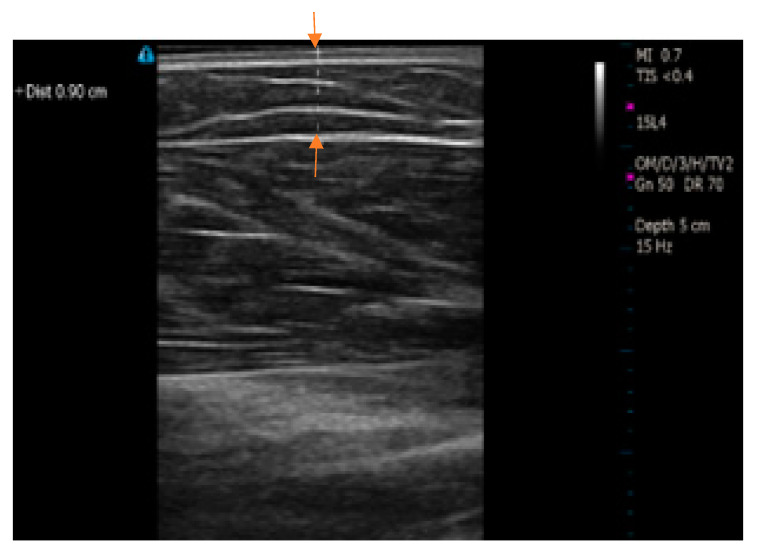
Ultrasound imaging for the measurement of subcutaneous fat thickness. Arrows show the measured skin-fat thickness.
